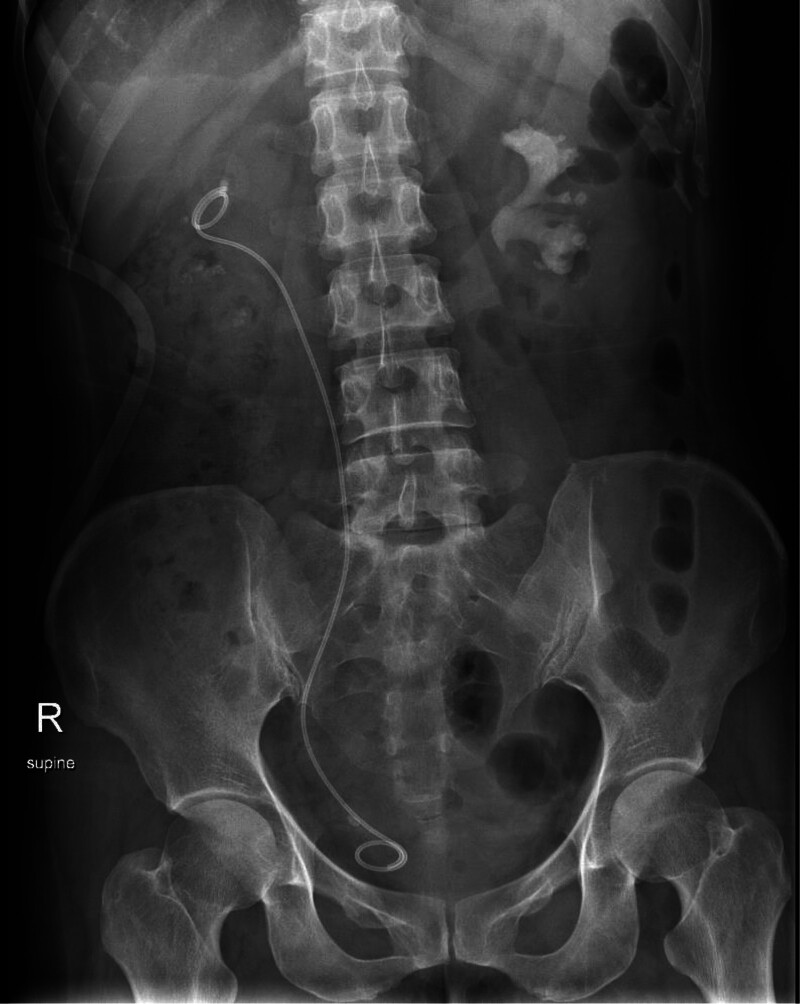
After the first surgery, an abdominal X-ray showed a significant reduction in the right-sided stones compared with before, and the right ureteral stent was in place.
